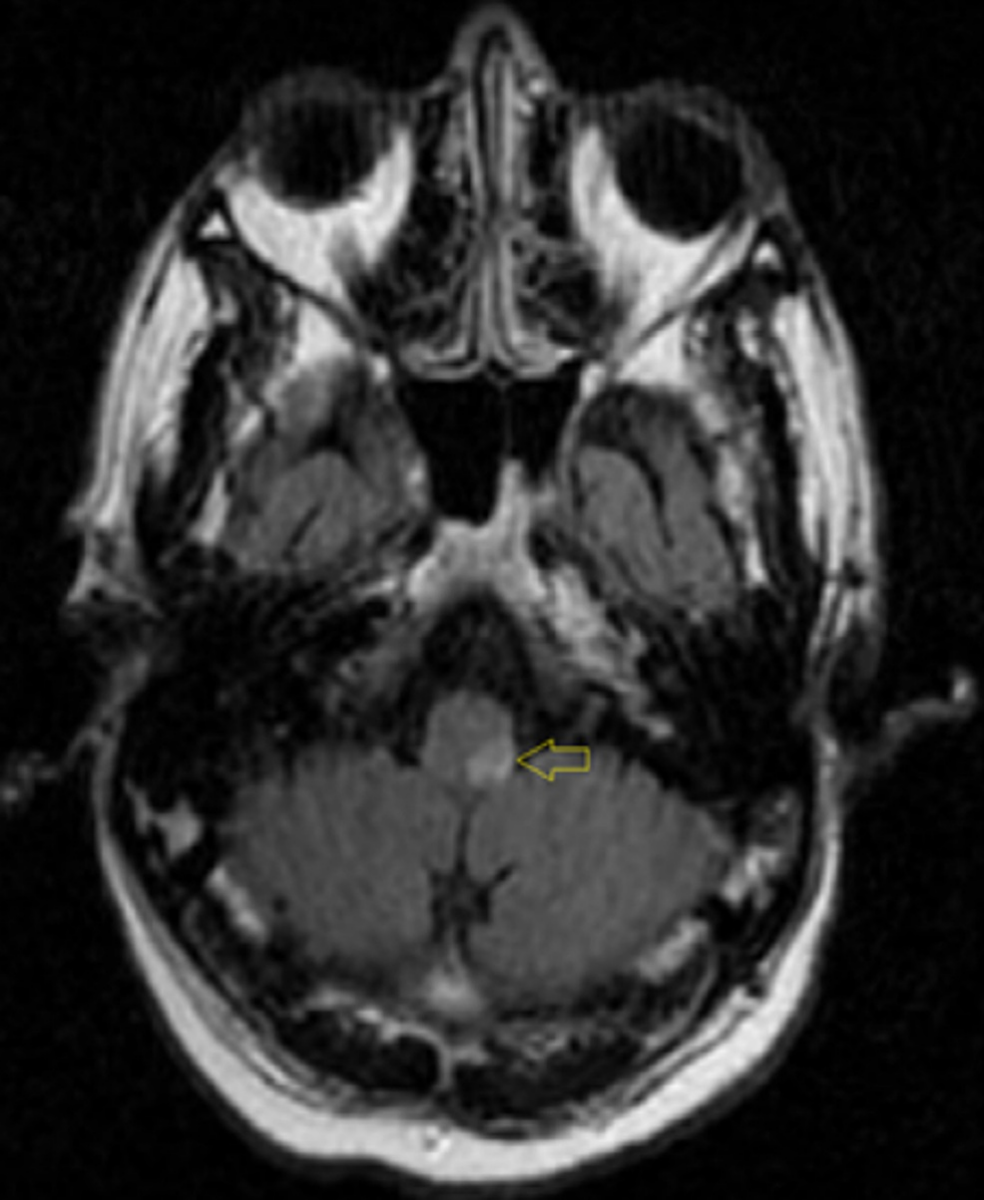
MRI of the brain showing a small focus of bright signal (hyperintensity) on T2-FLAIR T2-FLAIR: T2-weighted fluid-attenuated inversion recovery
